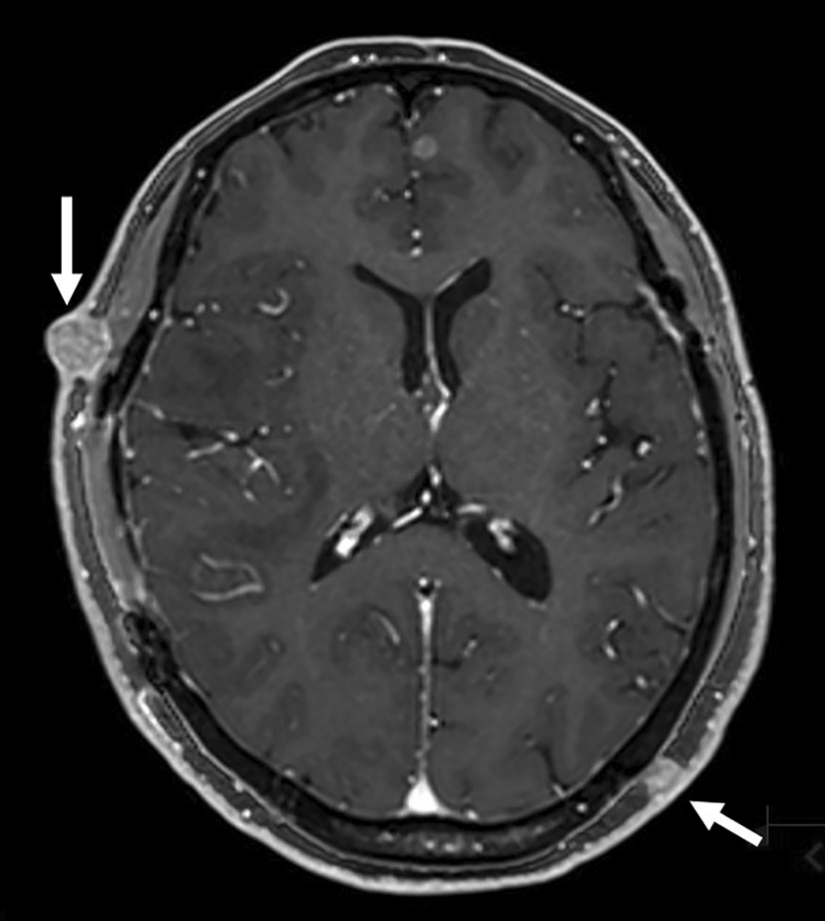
Cutaneous/subcutaneous metastases in a patient with esophageal cancer. A contrast-enhanced 3D fat-suppressed T1-weighted image shows homogeneously enhanced cutaneous/subcutaneous nodules (arrows)
